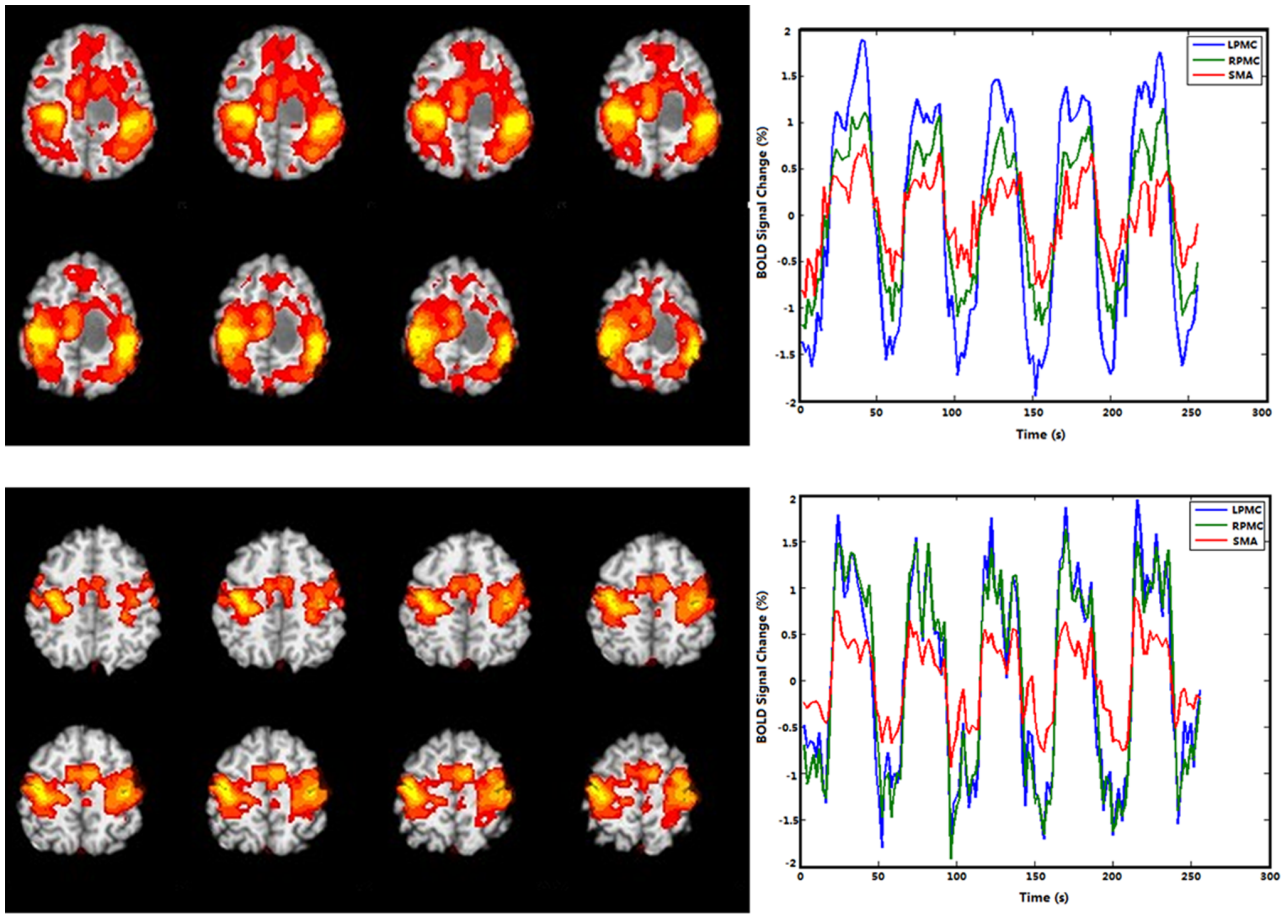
Example of BOLD functional magnetic resonance imaging activation maps and BOLD signal time courses in the LPMC, RPMC and SMA during a motor task in a single patient with glioma and a healthy control subject. BOLD, blood oxygen level dependent; LPMC, left primary motor cortex; RPMC, right primary motor cortex; SMA, supplementary motor area.
